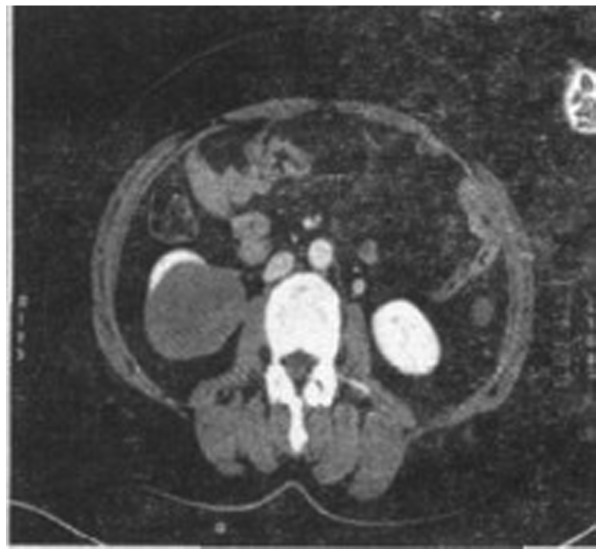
Venous phase computed tomography. The tumor showed marginal uneven enhancement in this phase.
